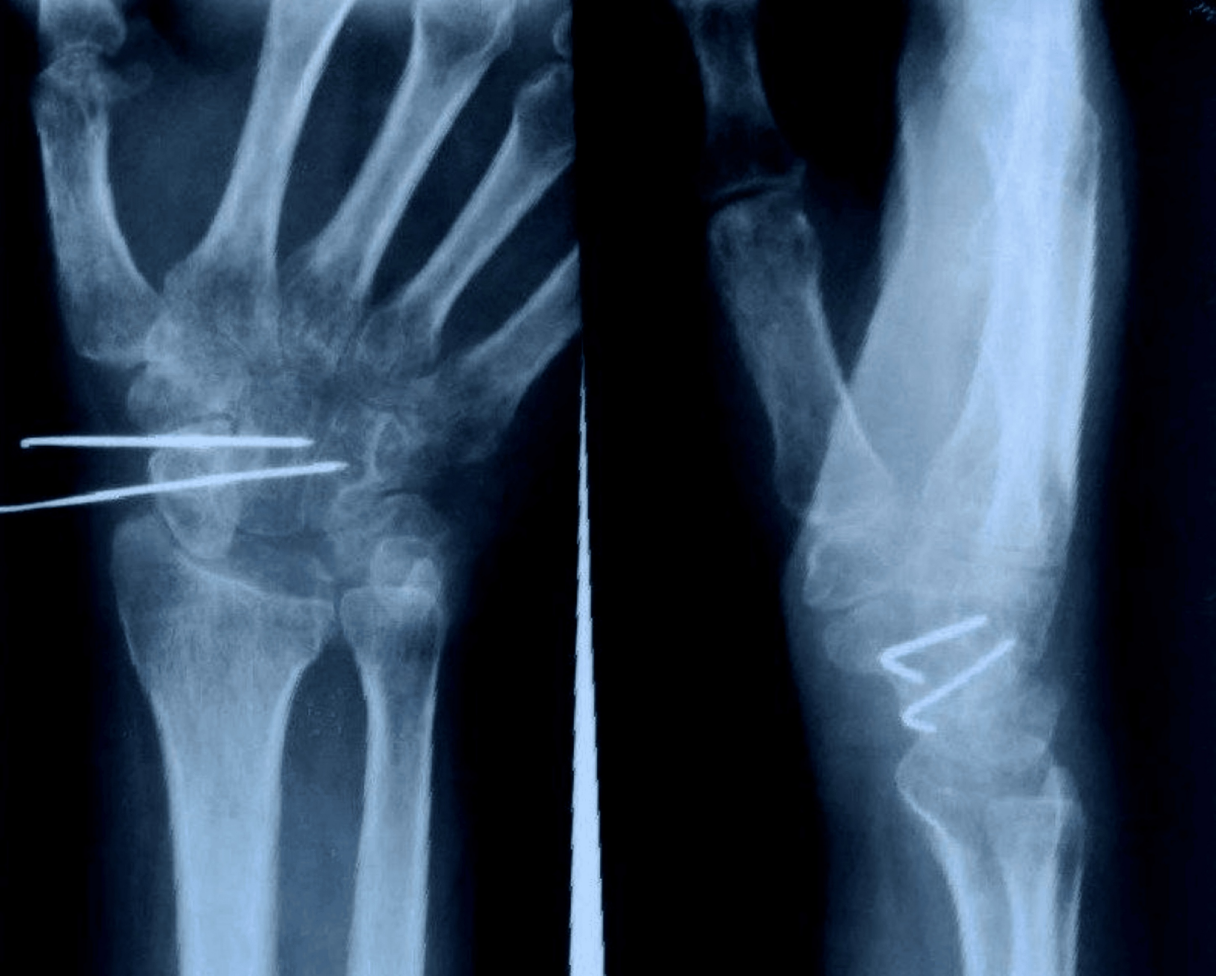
Postoperative radiographs showing Kirschner wires across the scaphocapitate joint. The lunate has been excised. The avulsion fracture of the triquetrum was left alone.
